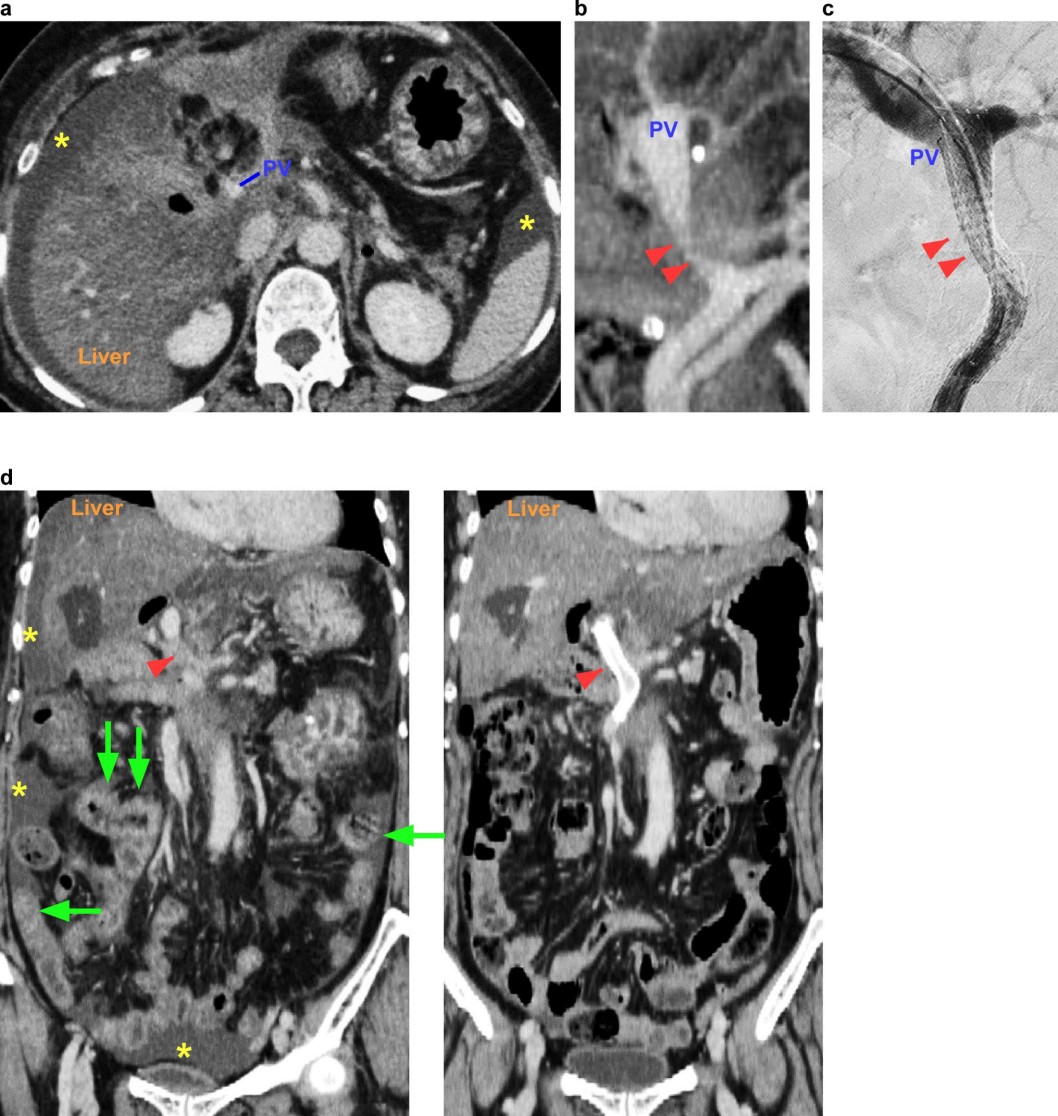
CT images on readmission and treatment for PV stenosis. a Liver CT values are decreased overall and there is ascites around the liver and spleen (_*_). b Maximum intensity projection image shows the PV stenosis (arrowhead). c PV stent is inserted via percutaneous transhepatic puncture. d Left: coronal CT image taken at the time of readmission showing ascites throughout and intestinal edema. Right: the same coronal CT view obtained 7 days after intervention shows that the ascites has disappeared and the intestinal edema has improved
